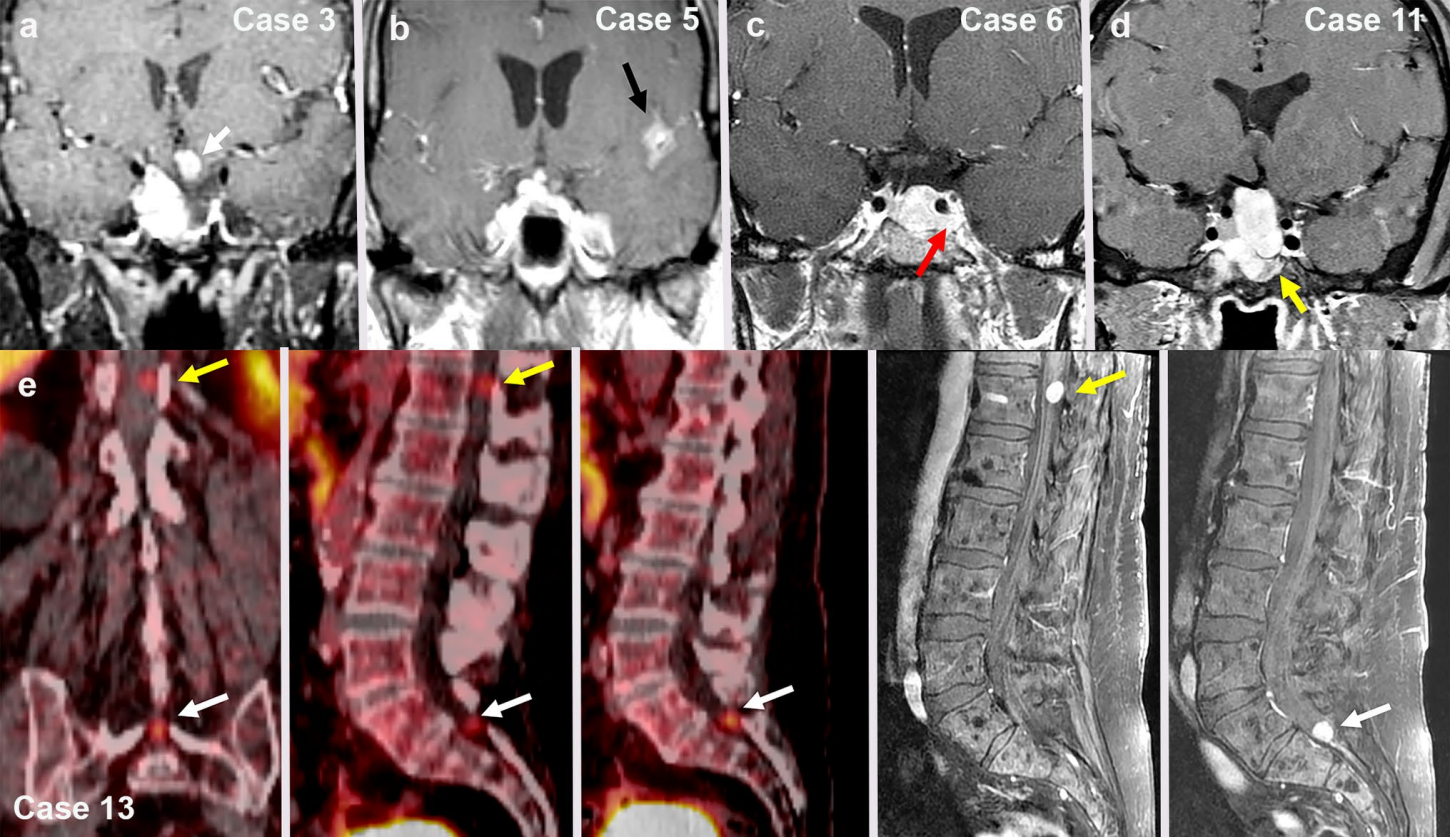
Examples of neuroimaging features on intracranial MRI (a, d) and spinal DOTATATE PET scan and MRI (e). a Separate infundibular enhancing nodule in patient 3 (white arrow). b Leptomeningeal enhancing nodule, representing CSF dissemination in patient 5 (black arrow). c Cavernous sinus invasion and expansion in patient 6 (red arrow). d Clival invasion in patient 11 (yellow arrow). e Two separate drop metastases representing CSF dissemination in case 13 (yellow and white arrows)
